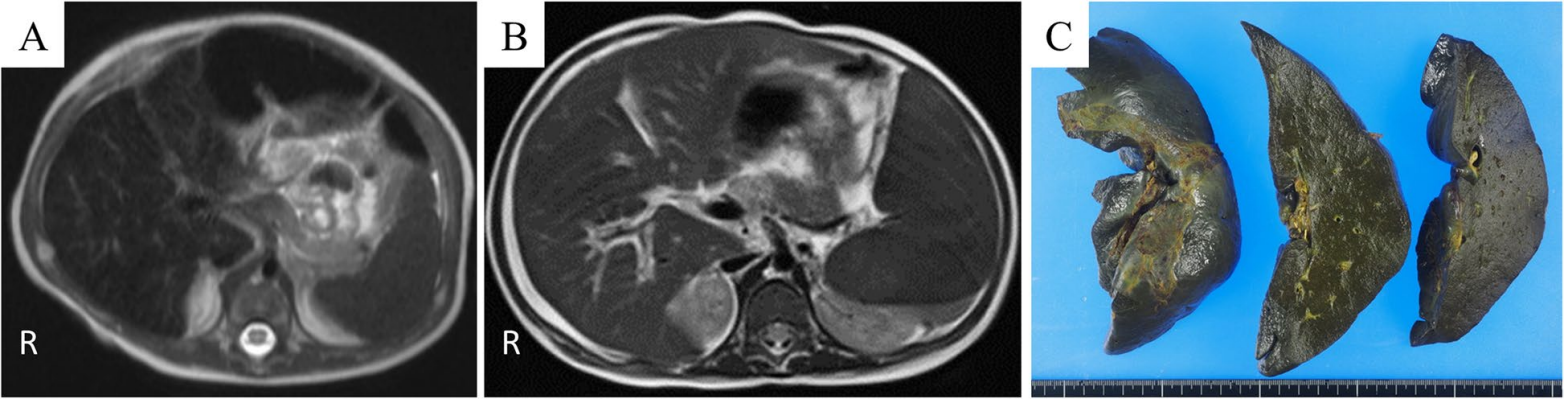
Magnetic resonance imaging and photograph of the liver. Coronal T2 weighted magnetic resonance images of the abdomen at 3 months of age (A) and 5 months of age (B), showing hepatosplenomegaly and diffuse low-intensity of liver, pancreas, spleen and spinal bone marrow due to iron deposition. (C) Gross photograph of resected liver, demonstrating a dark green cut surface
